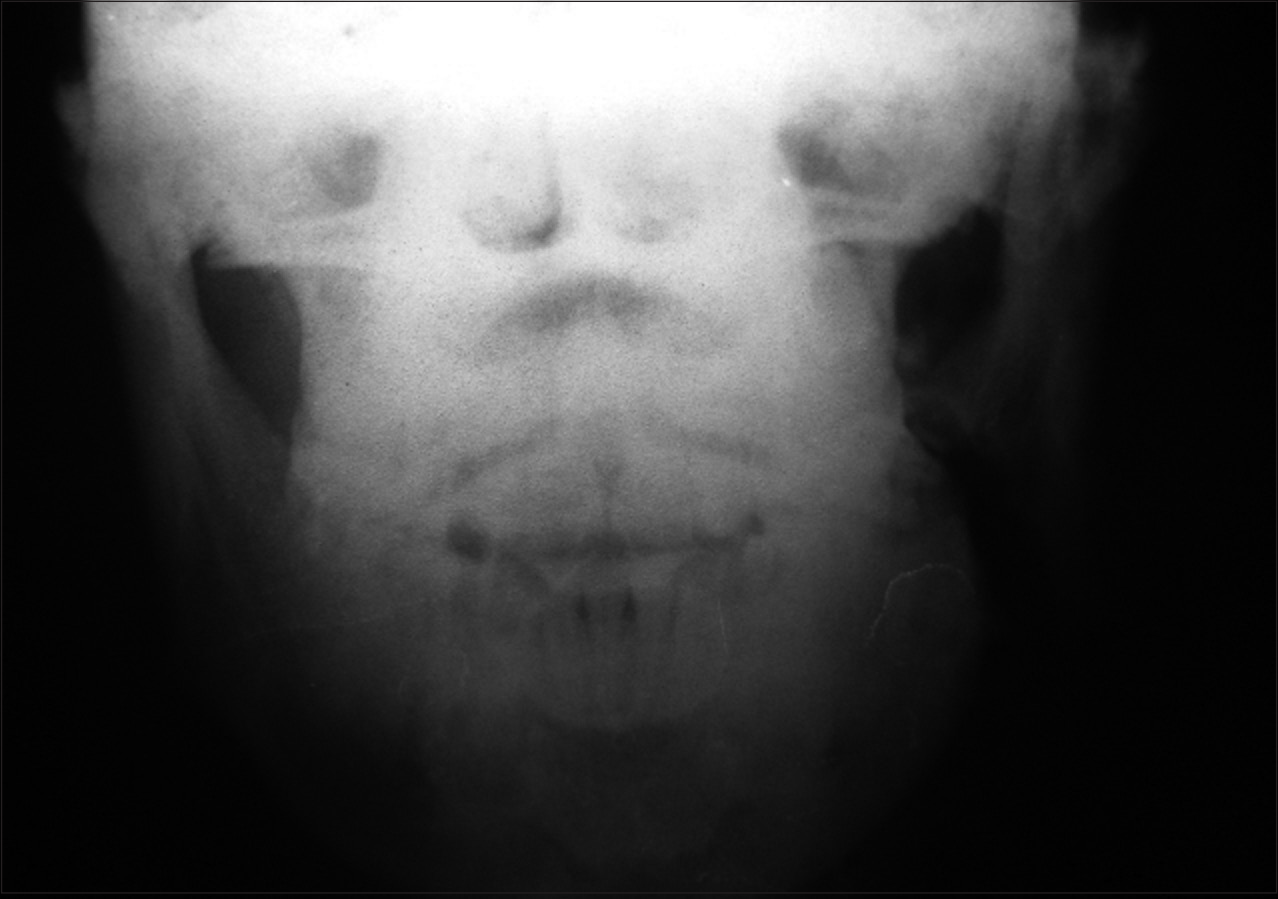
X-ray skull showing fracture of mandible left side
